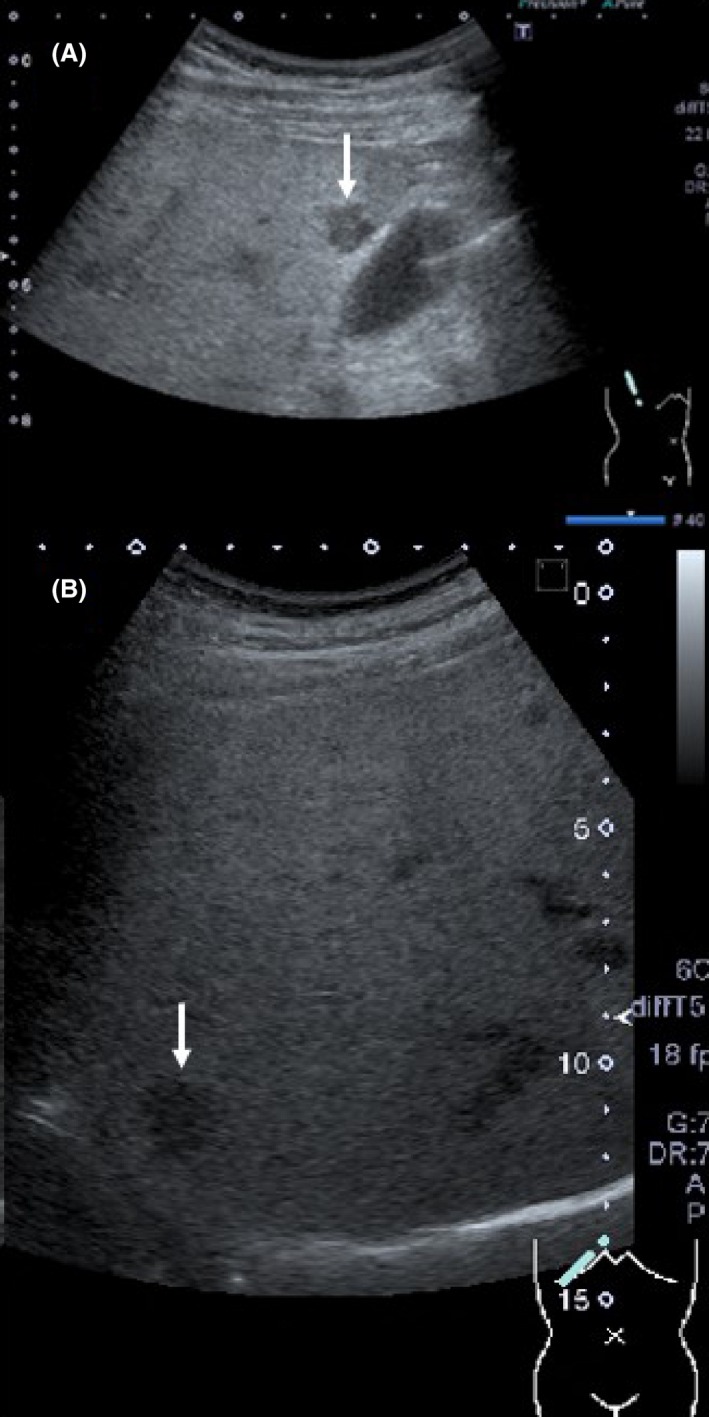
Ultrasonographic images of the liver on admission. (A) An irregularly shaped, 1‐cm‐diameter, low‐echoic focal lesion was observed in S5 adjacent to the gallbladder (white arrow). At least two other lesions showing similar characteristics and size were detected in S5; these lesions were not detected on contrast‐enhanced abdominal computed tomography. (B) A larger (2‐cm‐diameter) low‐echoic lesion was also observed in S6 (white arrow). This was the only lesion detected on contrast‐enhanced computed tomography of the abdomen.
